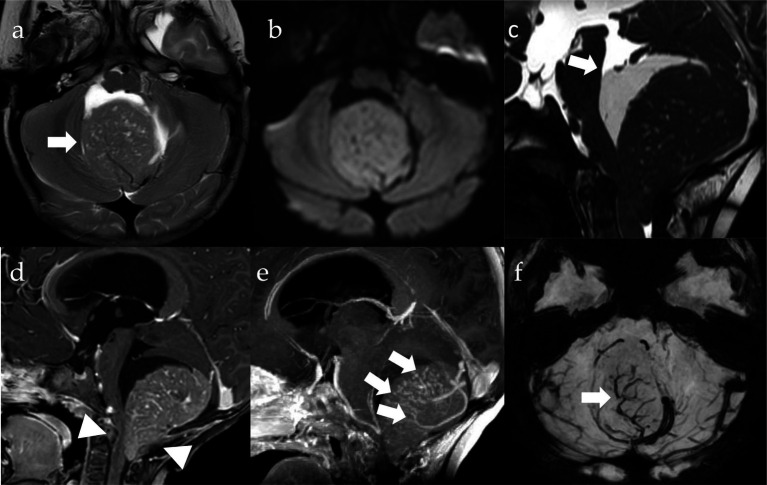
MRI imaging. Pre-operative axial T2w (A), DWI (diffusion weighted imaging, B) and SWI (susceptibility weighted imaging, F) images, sagittal CISS (three-dimensional constructive interference in steady state, C) Gd T1w (D) and MIP (maximum intensity projection, E) images. There is a well-circumscribed lesion in the posterior fossa (A, arrow), which is centered on the cisterna magna and pushing the vermis cranially, with growth into the fourth ventricle and extension through the foramen of Magendie onto the posterior aspect of the upper cervical cord (D, arrowheads). The tumor is isointense to the cerebellar cortex on T2 (A) and shows restriction of diffusivity (B) due to high cell density along with high nuclear-to-cytoplasmic ratio (B). There is significant contrast-enhancement (D) and intralesional vessels (E,F, arrows). Cystic components are appreciable and appear larger in the cranial portions of the lesion (arrow, D).
